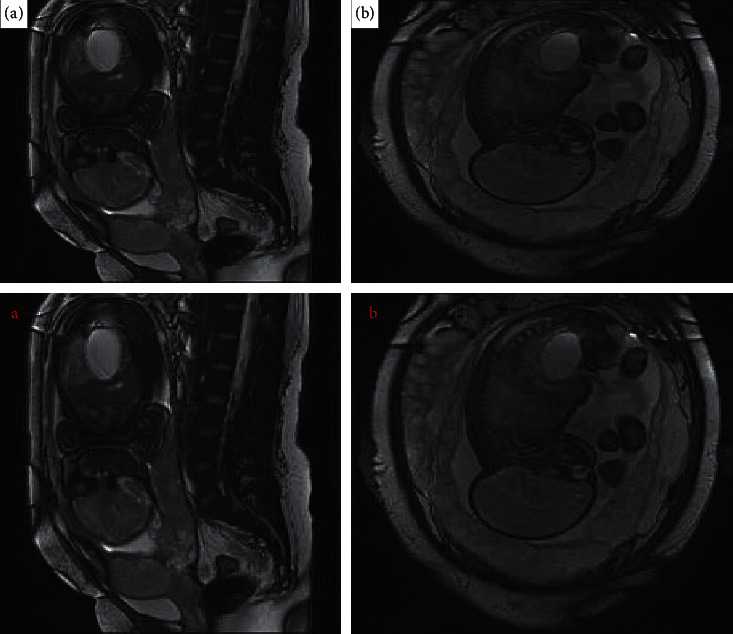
The MRI image of a marginal placenta previa case. (a) The sagittal plane. (b) The transverse plane.
